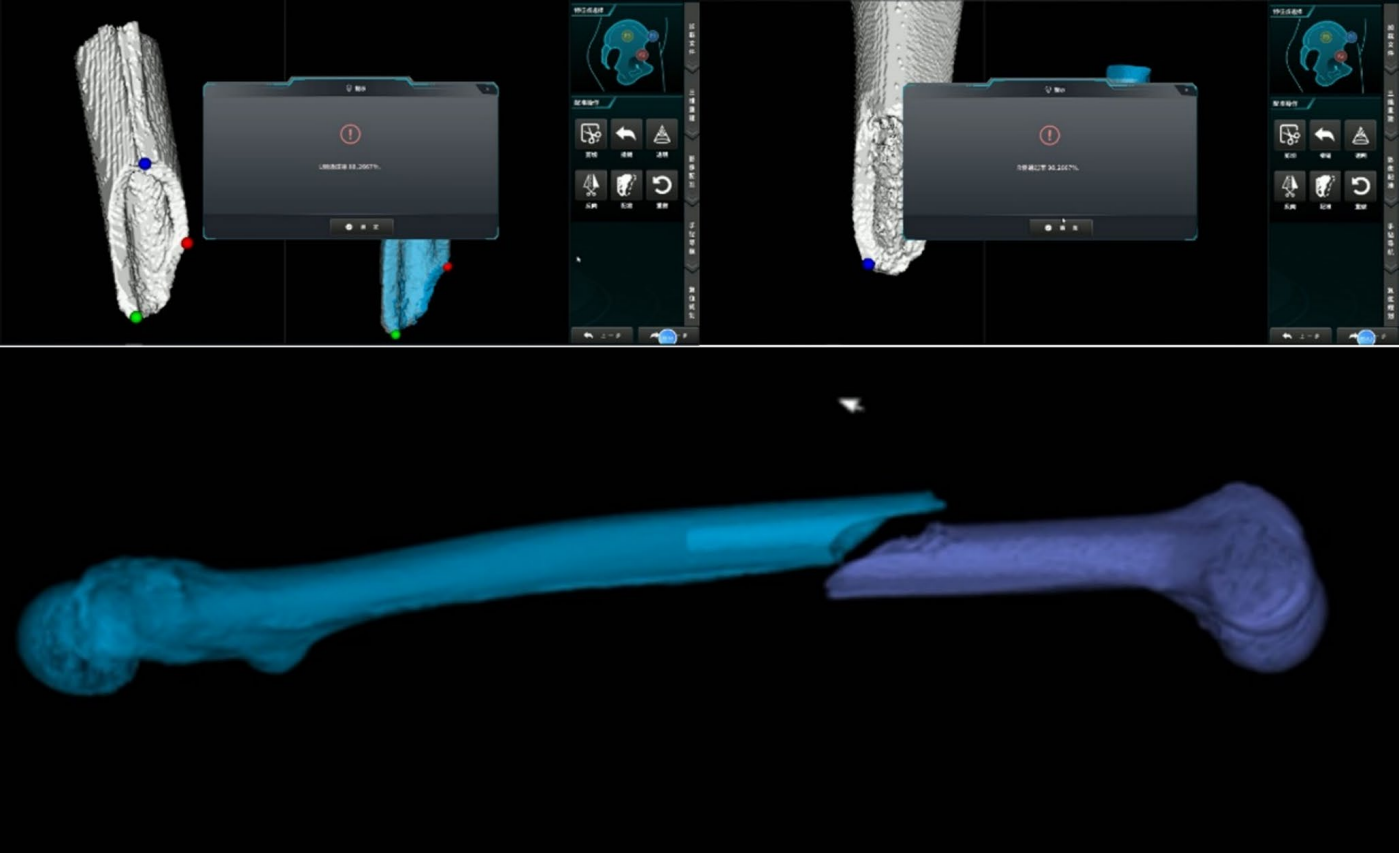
Registration of intraoperative CBCT and preoperative CT images for precise fracture alignment
